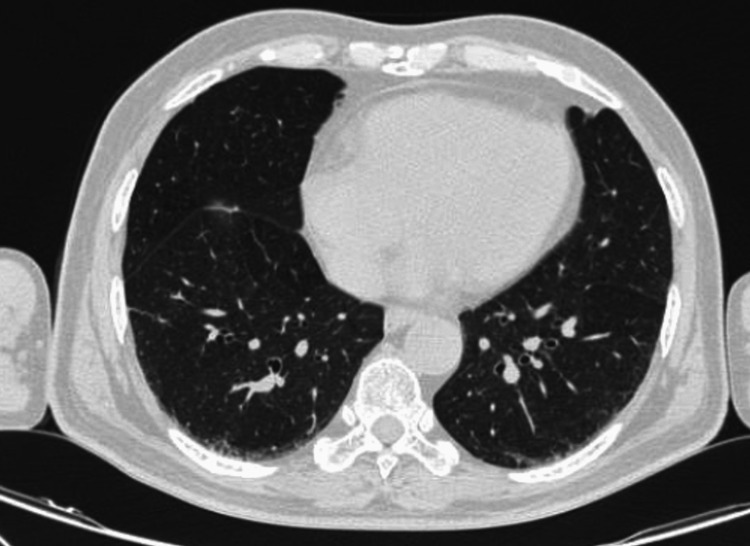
CT lung CT lung thin sections axial view showing no discrete lesions CT: computed tomography
